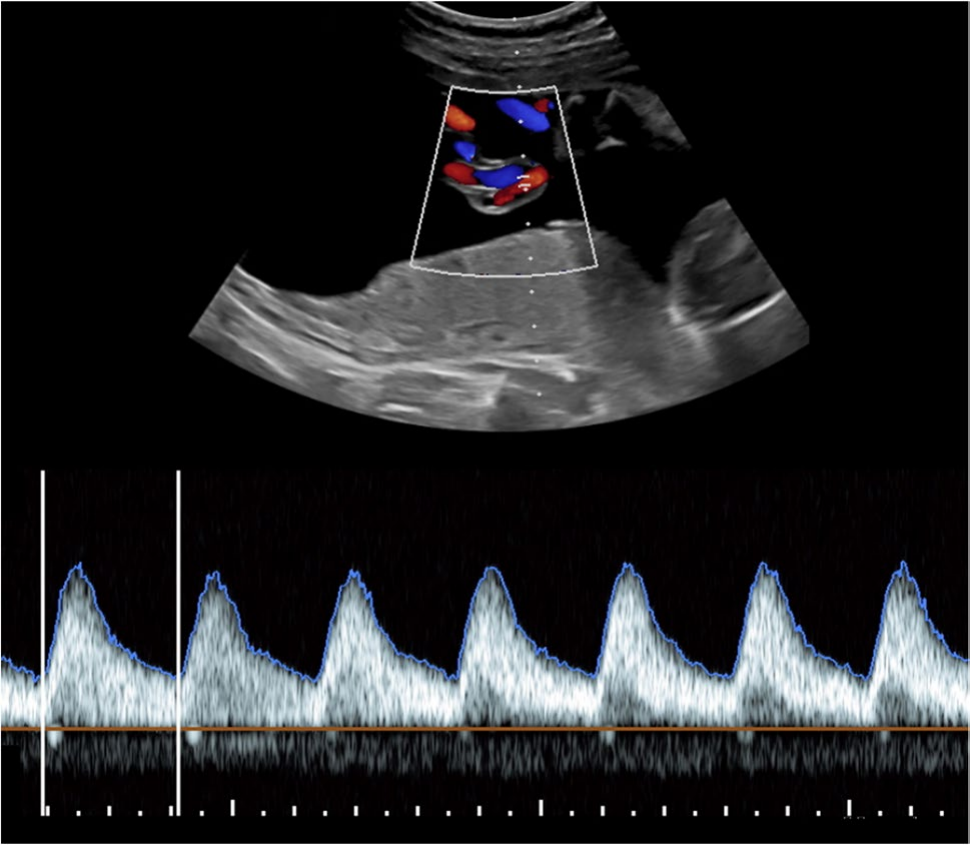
Case 11 at 30 + 0 weeks of gestation, CVPS 8 points (skin edema: 0 points, cardiothoracic area ratio = 0.28: 2 points, competent tricuspid and mitral valve: 2 points, normal arterial Doppler flow velocimetry: 2 points, normal venous Doppler flow velocimetry: 2 points)
